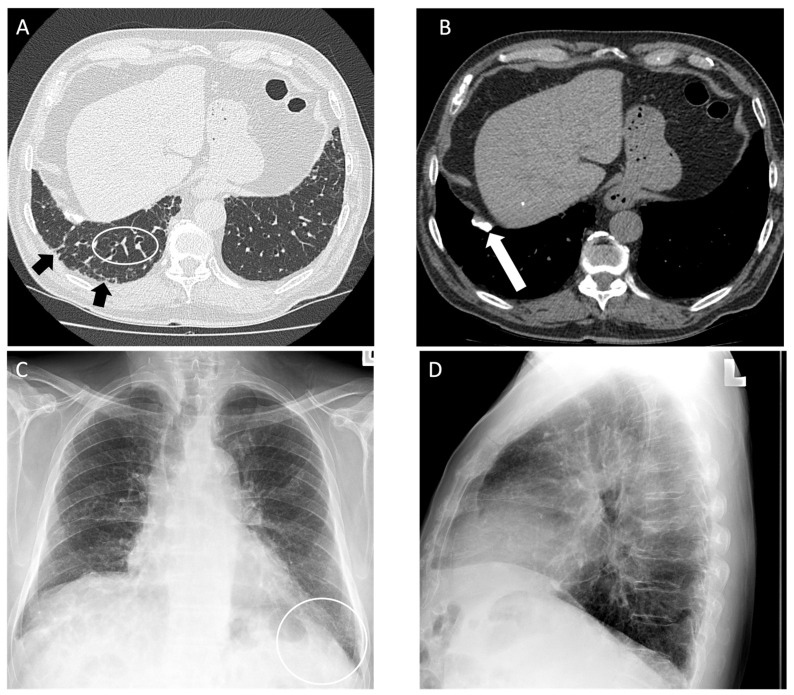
An 83-year-old male with 75 pack year smoking history and asbestos exposure from construction work, diagnosed with asbestosis. (A) Axial computed tomography image demonstrates subpleural reticulation (black arrows) and mild traction bronchiectasis (white circle) of right lung in keeping with mild asbestosis; (B) mediastinal windows demonstrate a calcified pleural plaque along the right diaphragm (white arrow); (C) posteroanterior and (D) lateral chest radiograph demonstrate mild reticular markings in the basal lung consistent with mild fibrosis, best appreciated in the left lateral costophrenic angle (white circle).
